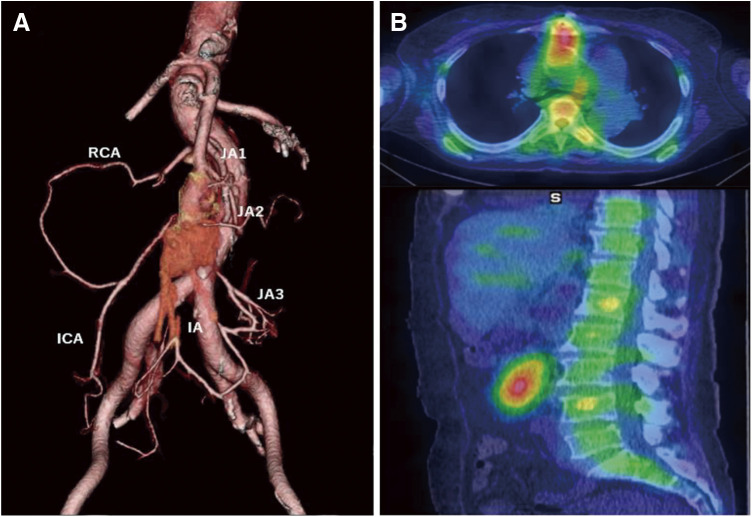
Fig. 1 (A) The SMA aneurysm was located 60 mm distal to the origin of the SMA and branched out as the third JA, the ICA, and IAs. The second JA and the right colic artery originated from the proximal neck of the SMA aneurysm. (B) Gallium citrate scintigraphy demonstrated an intense uptake in the prosthetic graft and SMA aneurysm. SMA: superior mesenteric artery; JA1: the first jejunal artery; JA2: the second jejunal artery; JA3: the third jejunal artery; RCA: right colic artery; ICA: ileocolic artery; IA: ileal artery
